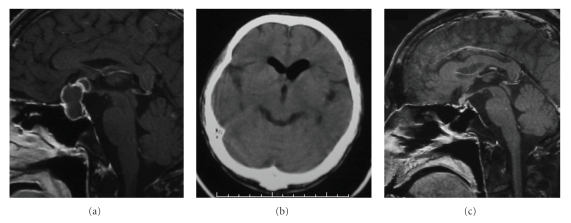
(a) Preoperative sagittal contrast-enhanced T1-weighted MRI demonstrating a ring-enhancing mass in the sella extending to the suprasellar legion. (b) Brain CT scan performed when the CSF rhinorrhea occurred (19 days after the operation) showing pneumocephalus. (c) MRI scan performed at the same time revealing no recurrence of abscess.
